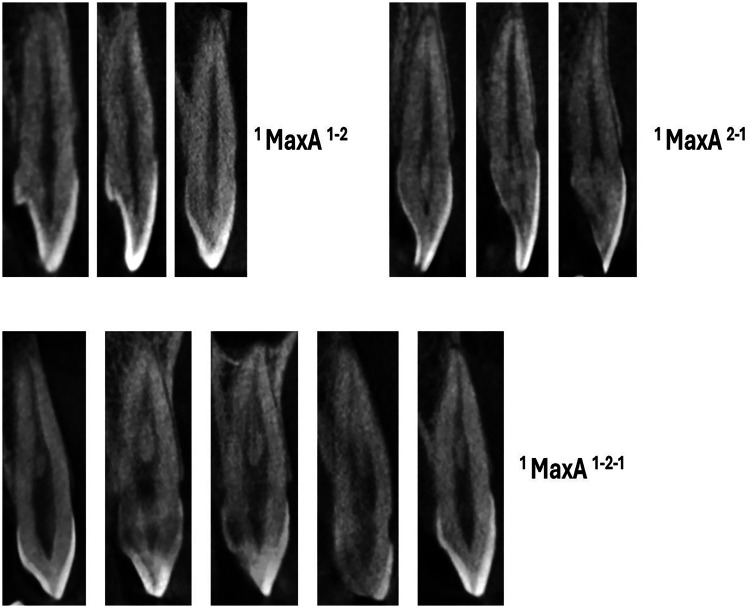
CBCT sagittal view of maxillary anteriors with two and three code classifications (maxA-maxillary anteriors).
